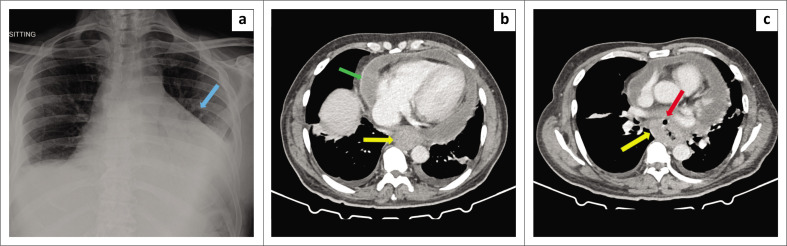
Chest X-ray (a) from day 9 (with clinical and echocardiographic cardiac tamponade), showing traverse, crisp cardiomegaly in keeping with pericardial effusion (blue arrow). Computed tomography (CT) scan of the chest in (b) and (c) showing rim enhancing pericardial effusion (green arrow), circumferencial thickening of the oesophagus (yellow arrows) and an anterior oesophageal air locule suggestive of mural breech into the pericardium (red arrow).
